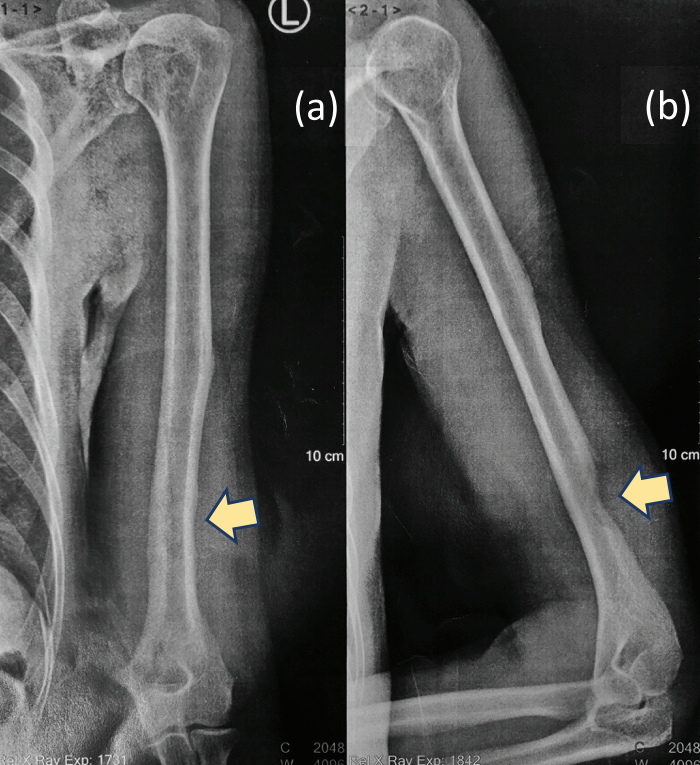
(a): Anteroposterior and (b): lateral radiographs of the left arm showing erosion of the posterolateral cortex of the distal humerus (yellow arrows).
